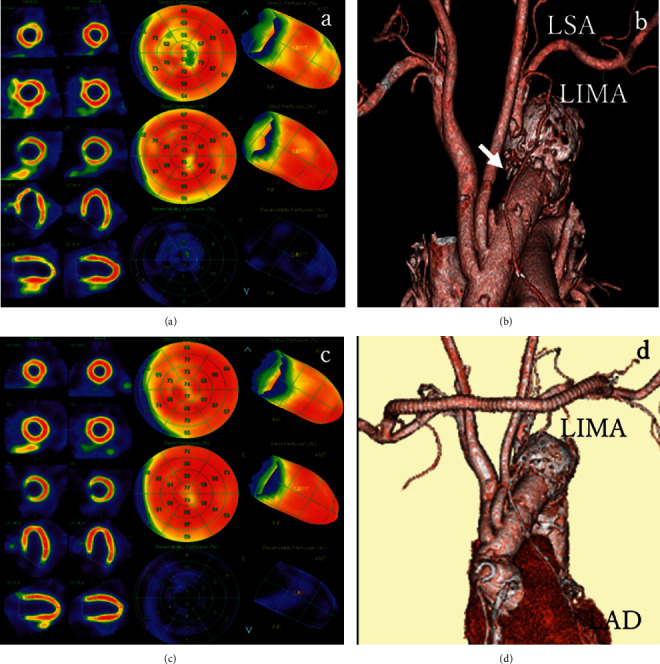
Myocardial scintigraphy and contrast-enhanced CT. Stress myocardial scintigraphy 7 days after the PCI procedure showed anteroseptal ischemia (a). Contrast-enhanced CT confirmed left subclavian artery occlusion at the anastomosed site (arrow) (b). Stress myocardial scintigraphy 3 days after axillo-axillary artery bypass surgery revealed improvement of myocardial ischemia (c). Contrast-enhanced CT 21 days postoperatively showed patency of the bypass graft (d). LAD: left anterior descending artery; LIMA: left internal mammary artery; LSA: left subclavian artery.
